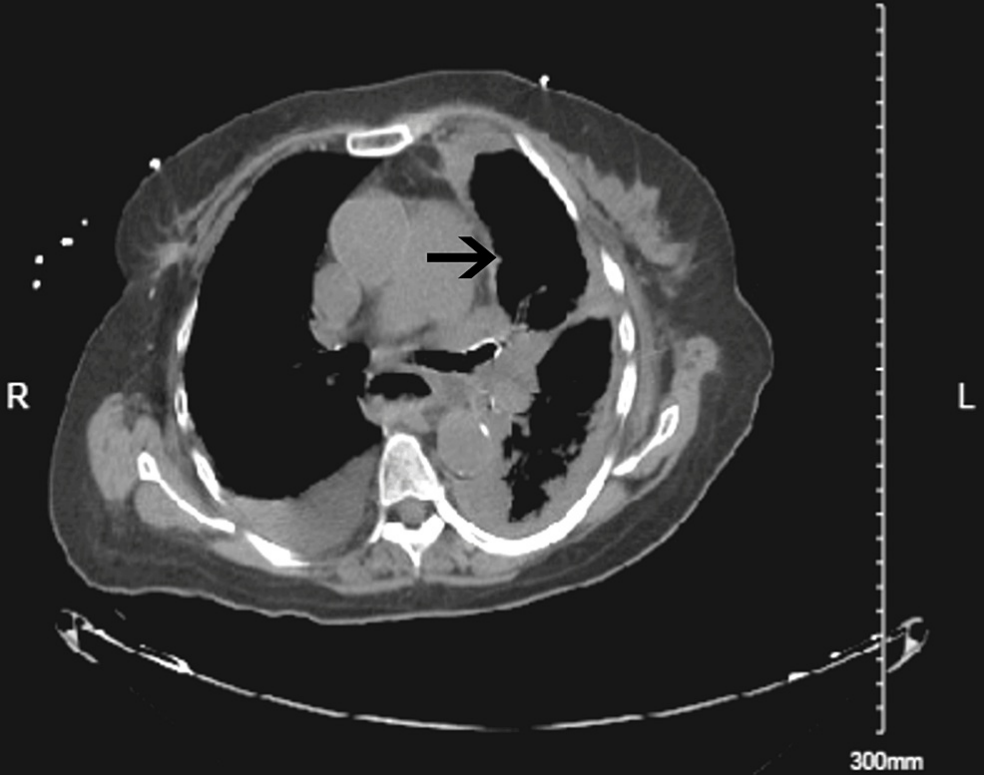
Repeat CT of the chest post surgery showing improvement in left-sided pleural effusion.
